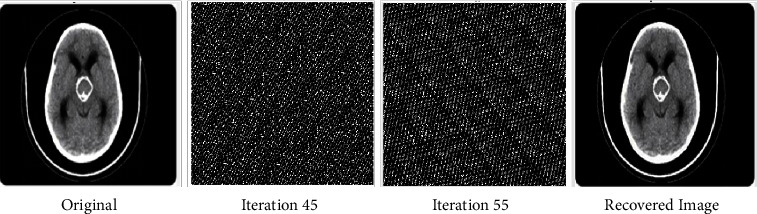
Before and after encryption of ACM (CT brain).
